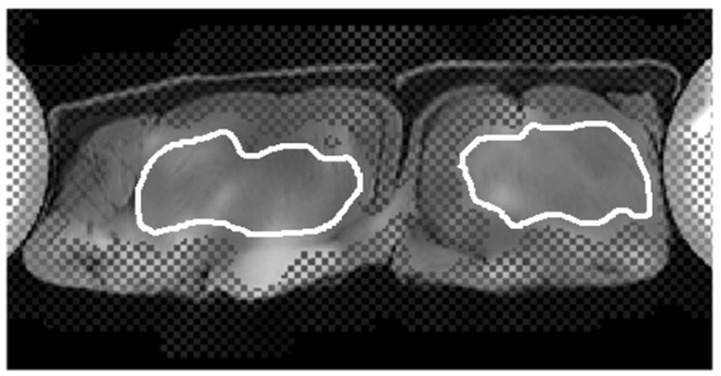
A fusion image of the T1-weighted DIXON water-only image. The post-calibration confidence map showed large regions (>50% of muscle size) with acceptable confidence in the two muscles, and two ROIs were placed on the two muscles for stiffness measurement.
